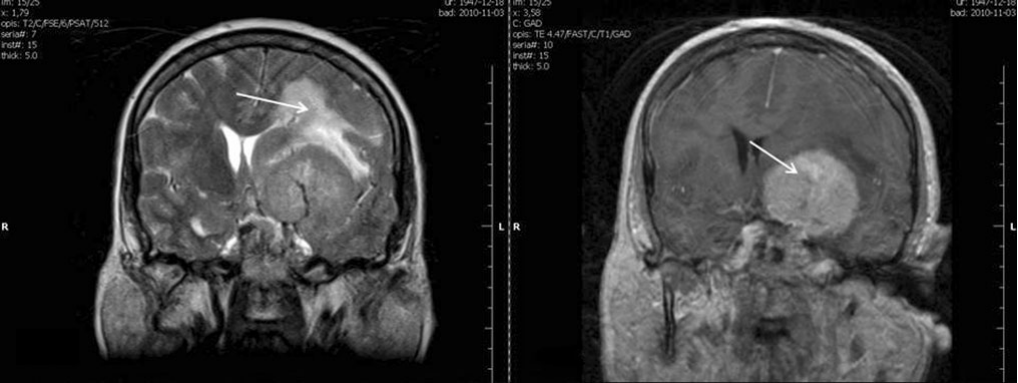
MRI scans showing peritumoral brain edema associated with meningioma, estimated (using Steinhoff classification) as grade II
